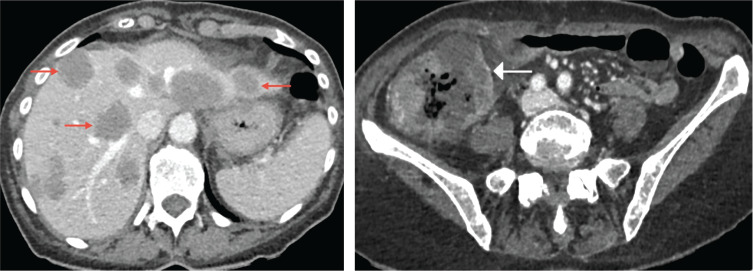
First contrast-enhanced CT scan (July 9, 2025) showing a thickened ascending colon (white arrow) and hypodense hepatic lesions without gas (red arrows).
